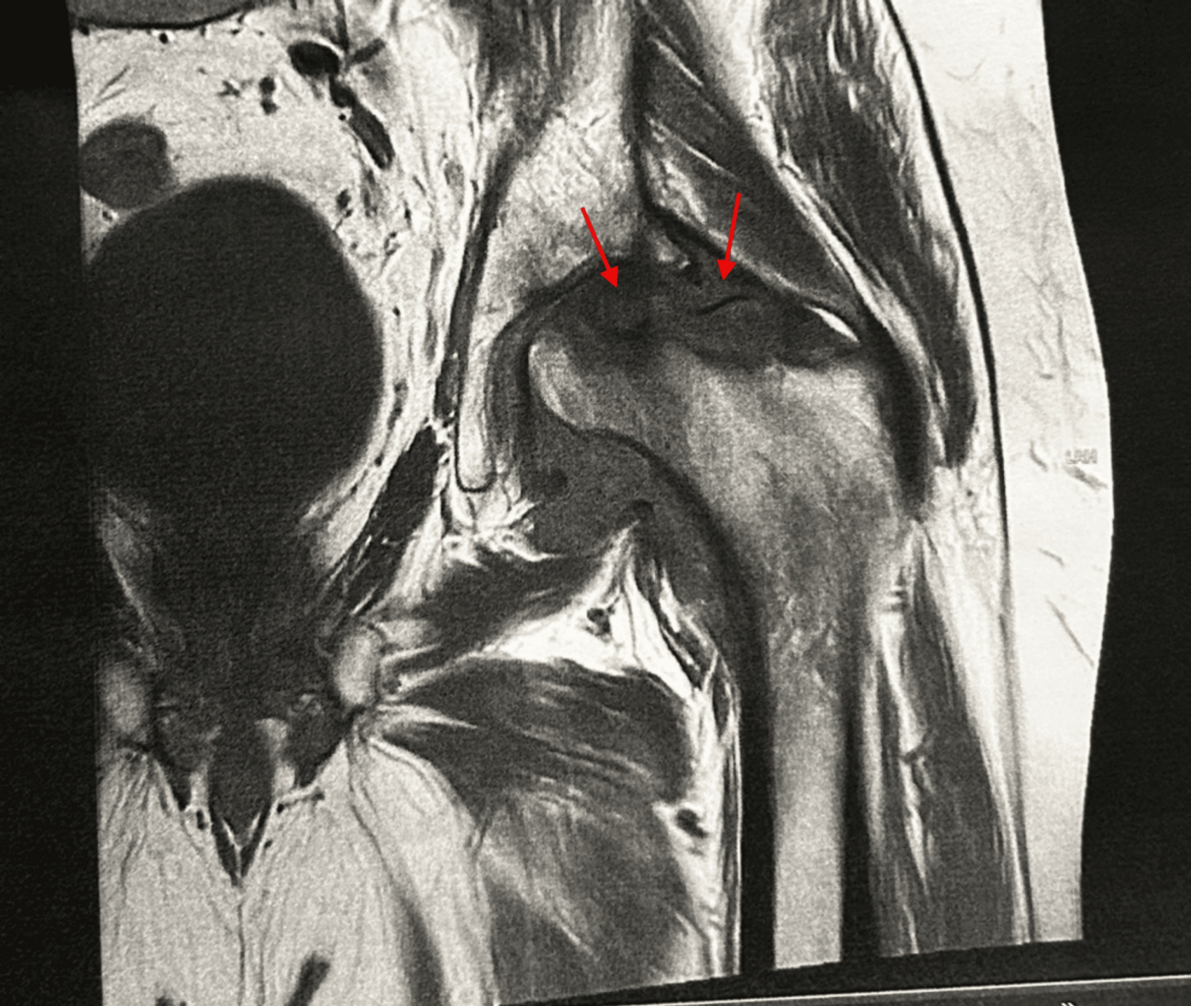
Magnetic resonance imaging of the left hip without contrast demonstrating crescentic fracture line
